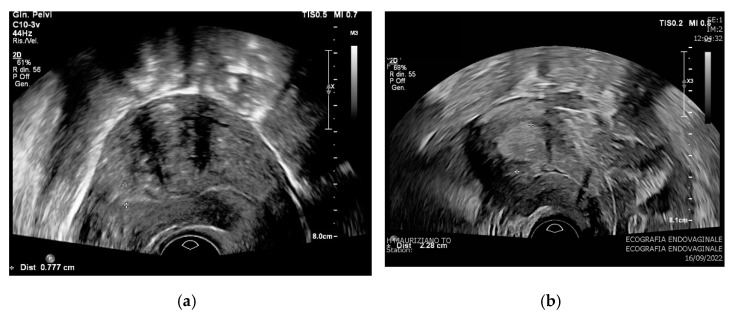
Transvaginal ultrasound (TVUS), endometrial thickness of two patients with a preoperative di-agnosis of atypical endometrial hyperplasia (AEH). (a) TVUS: 7.7 mm of endometrial thickness with a posterior leiomyoma of the uterus, postoperative diagnosis of AEH. (b) TVUS: 22.8 mm of en-dometrial thickness, postoperative diagnosis of endometrial cancer pT1a G2.
